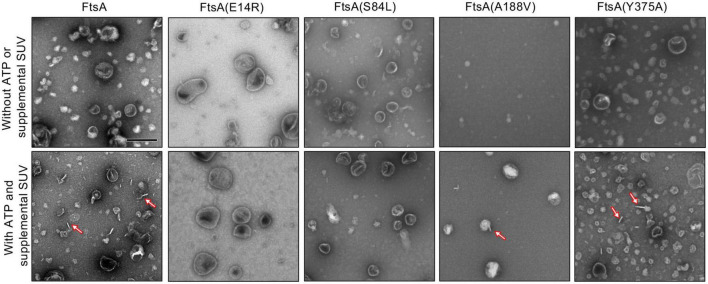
FtsA promotes vesicle tubulation with ATP. FtsA wild type and mutant proteins, FtsA(E14R), FtsA(S84L), FtsA(A188V), FtsA(Y375A), (4 μM) were incubated in the presence and absence of ATP (4 mM) and SUV’s (250 μg ml^– 1^) and visualized by TEM. Arrows indicate tubulated liposomes. Scale bar is 200 nm.
